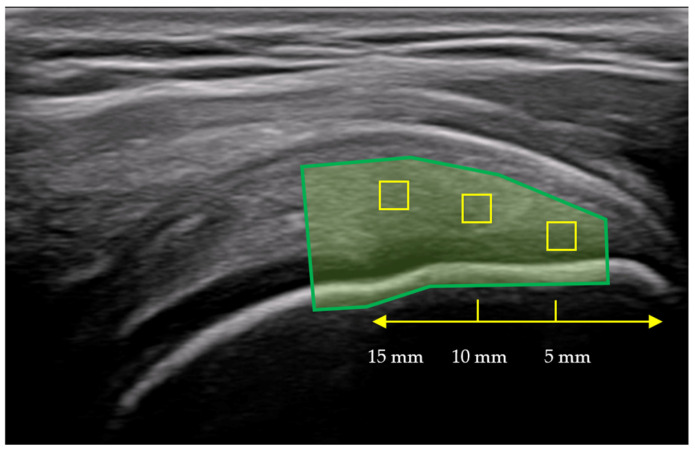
Ultrasound B-mode of the supraspinatus tendon with reference points for the measurement of peak spatial frequency radius (PSFR) at 5 mm, 10 mm, and 15 mm distances from the most hyperechogenic point at the greater tuberosity.
